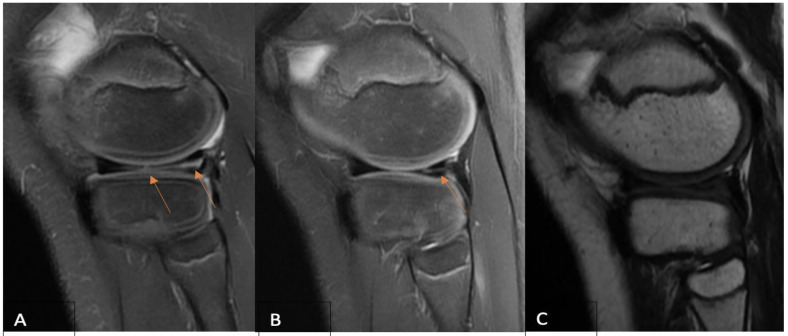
(A) A preoperative sagittal PD-FSE, FS MR imaging of an 11-year-old boy reveals (arrows) a complex (radial + longitudinal) type of lateral meniscus tear. Sagittal PD-FSE, FS (B), and T2-weighted FSE (C) MR images obtained 12 months after surgery, where sagittal PD-FSE, FS (B) indicates (arrow) a partially healed lateral meniscus with persisting intrameniscal signal changes that do not extend into the joint space, and sagittal T2-weighted FSE (C) shows no signal changes—MRI grade 2 due to Crues and Stroller grading. MR—magnetic resonance; FSE—fast spin echo; FS—fat saturation.
